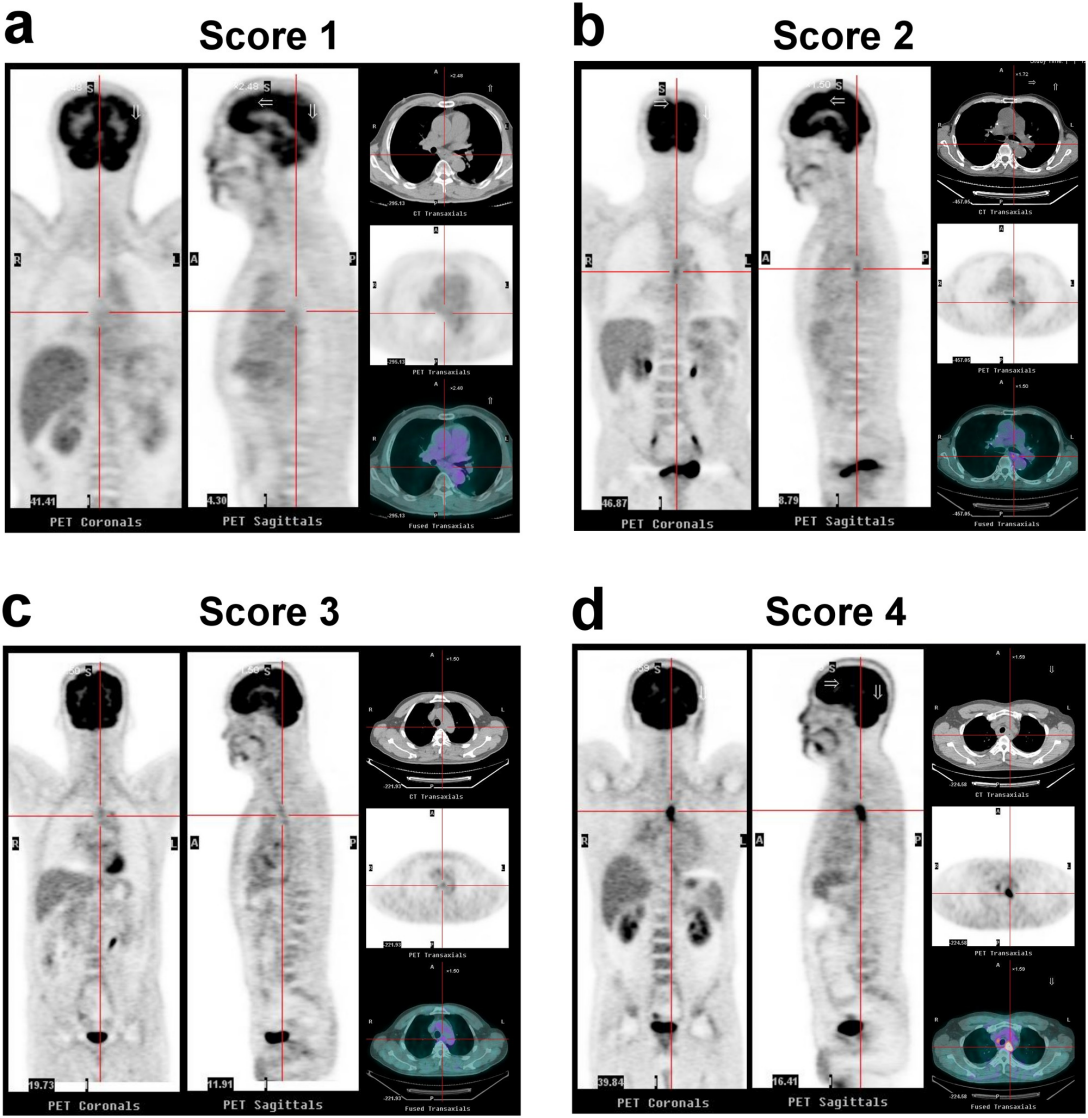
FDG PET/CT transaxial and sagittal images of four representative patients with Qual_4PS_ scores of 1–4. (a) Score 1: no detectable focal uptake; (b) Score 2: focal FDG uptake greater than that in the surrounding tissue or in the mediastinal blood pool, but not greater than that of the liver; (c) Score 3: diffuse FDG uptake greater than that in the mediastinal blood pool up to marginally greater than that of the liver, and suggestive of esophagitis; (d) Score 4: focal FDG uptake substantially greater than that of liver.
